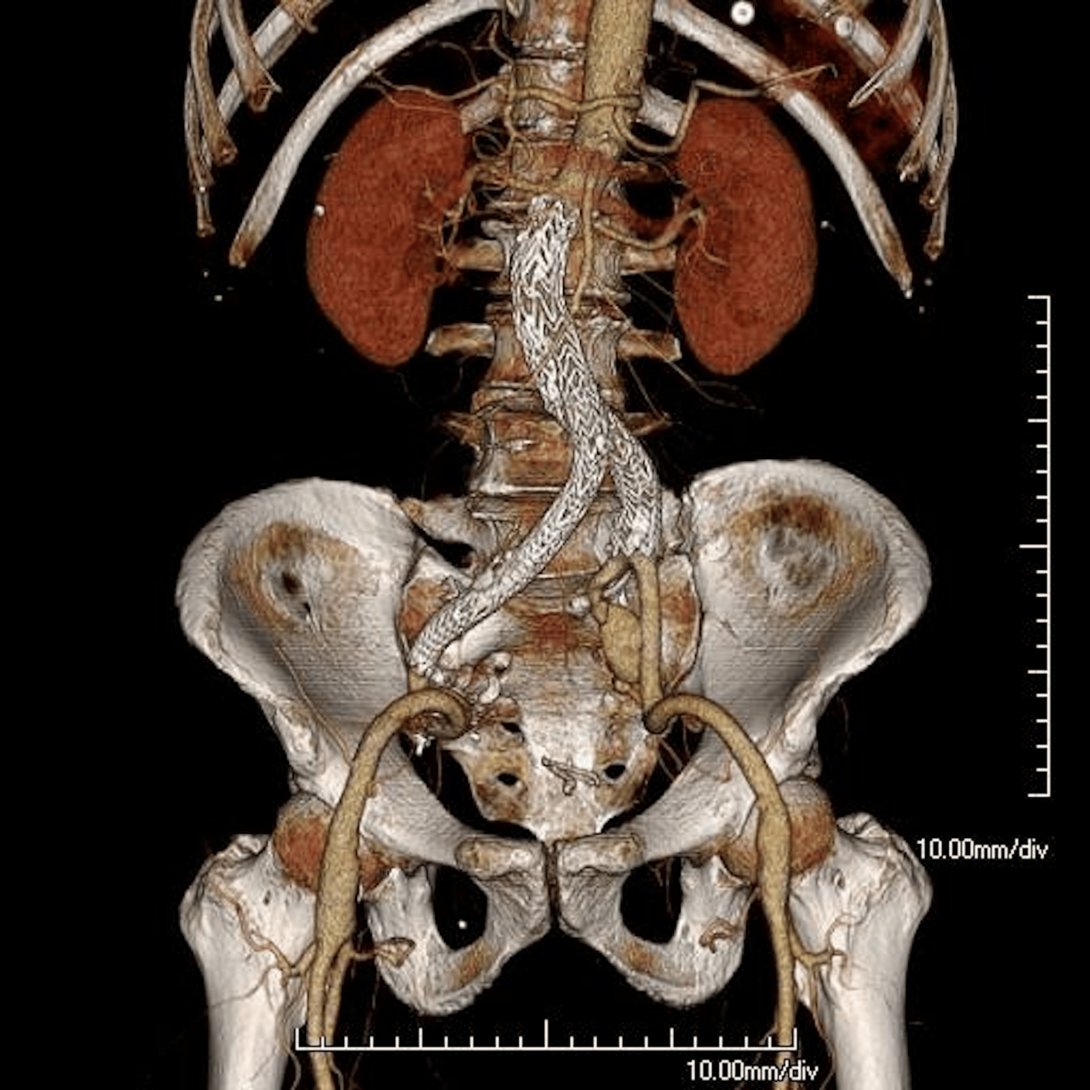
Volume-rendered reconstruction of postoperative CTA CTA demonstrates full expansion of the proximal endograft five days after the second procedure. CTA, CT angiography
